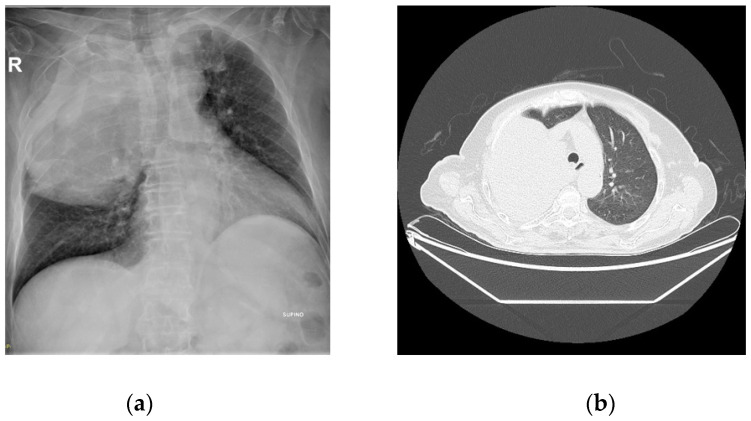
Radiographic images. (a) RX (X-Ray) and (b) CT scan show a large mass, with a maximum diameter of 15 cm, which almost entirely occupies the right lung and displaces the mediastinal structures contralaterally.
